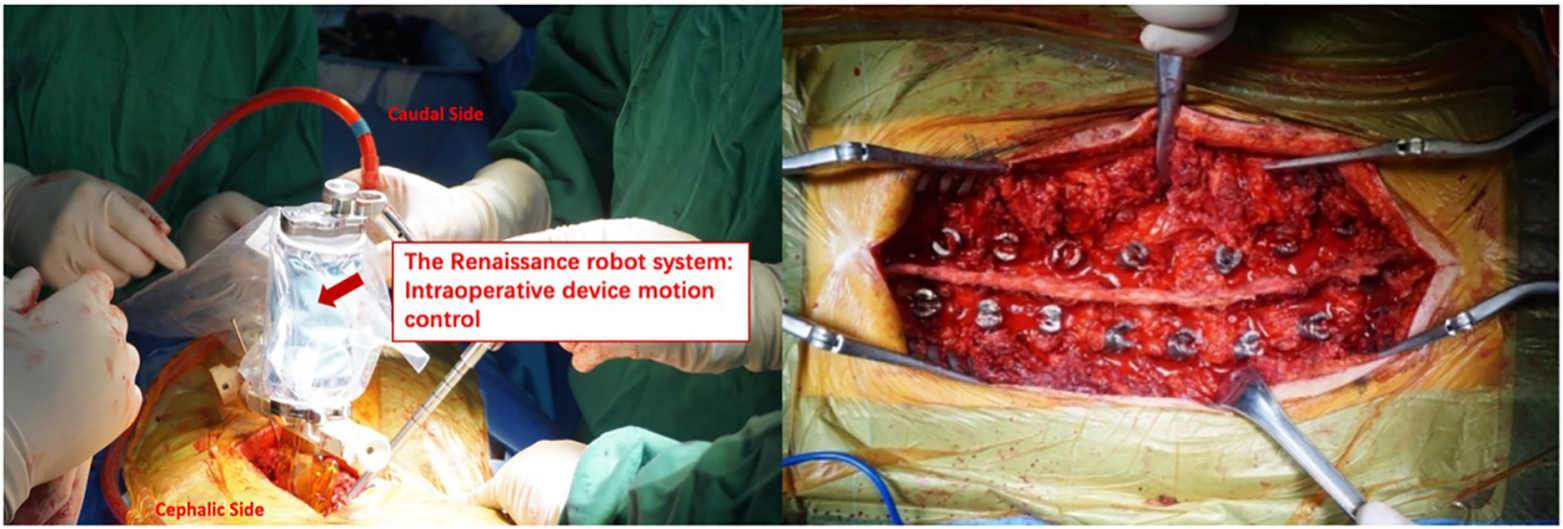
The controllable device of Renaissance robot system moves to planned screw trajectory position and direction on the basis of preoperative CT image, then pedicle screws were inserted according to the settled trajectory as drilled pathway.
